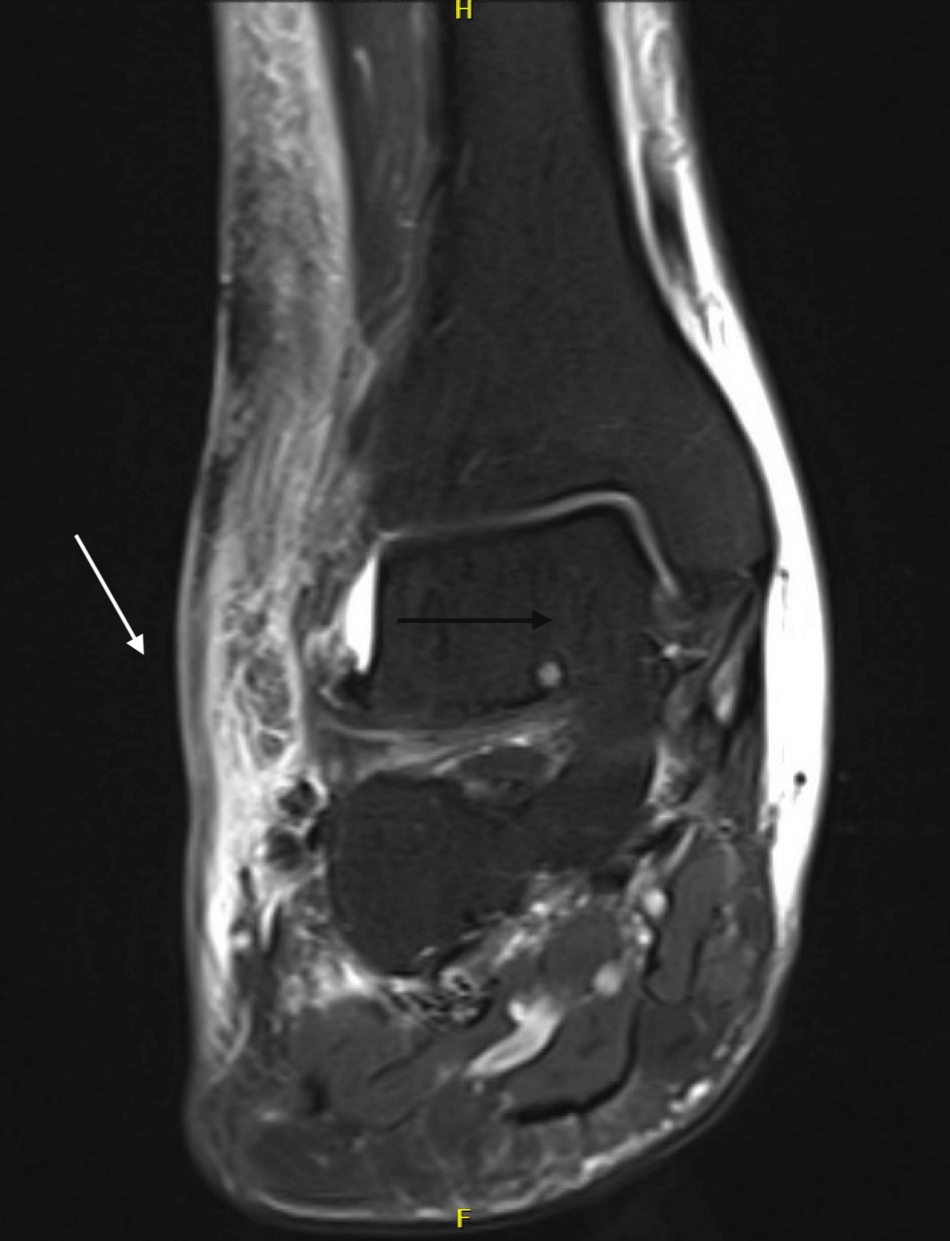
Coronal view of right ankle MRI without contrast showing superficial soft tissue edema and ulceration along the lateral ankle without osteomyelitis.
